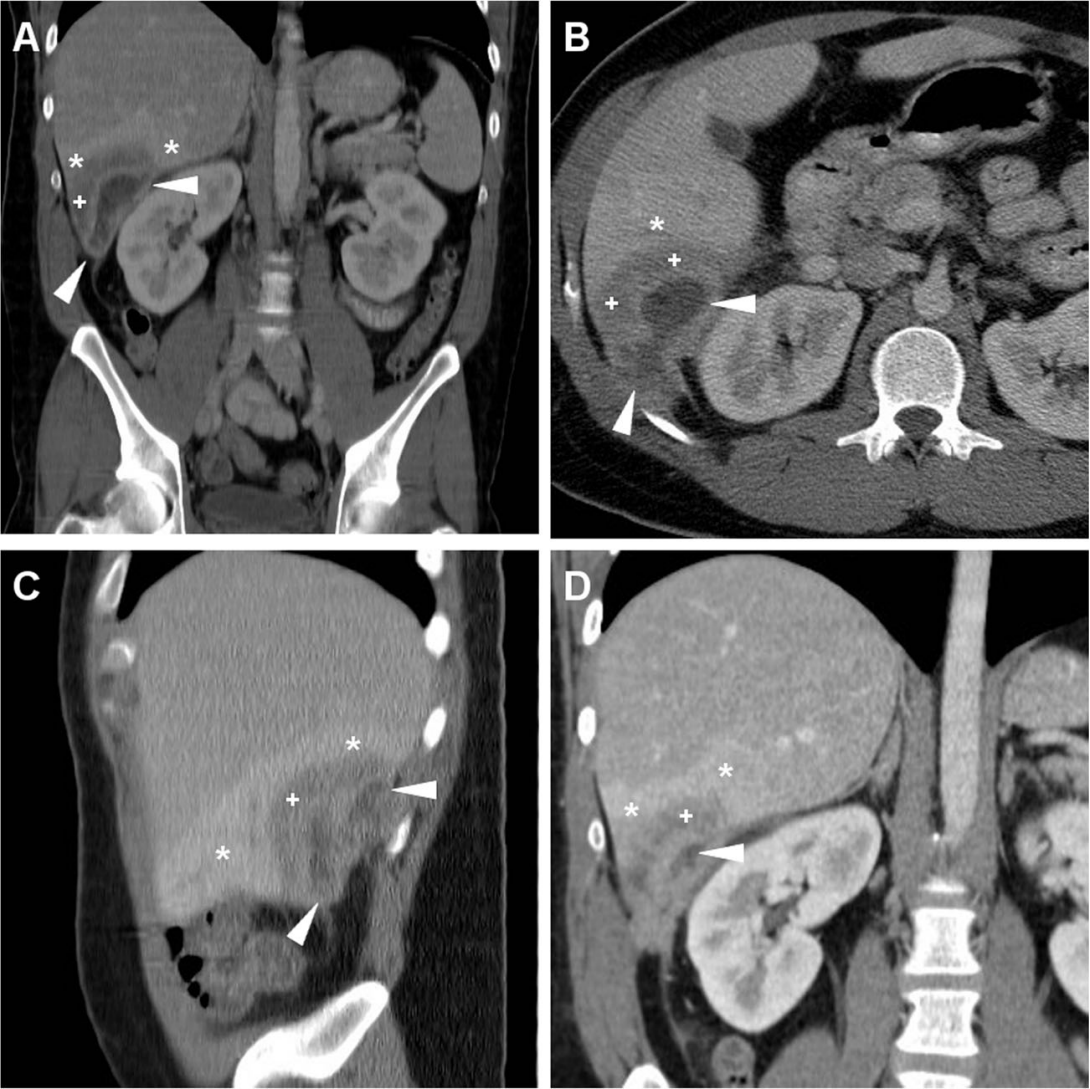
A 24-year-old male presented with mild fever, right lower quadrant pain and absent peritoneal irritation for a week, 3 months after LA for AA. Following ultrasound detection of a poorly defined hypoechoic lesion in the right liver lobe, multiplanar images from contrast-enhanced MDCT (a–c) showed a bilobated abscess in the sixth liver segment, with a strongly enhancing rim (*arrowheads*) and hypodense centre, surrounded by hypoattenuating oedema (+) and hyperenhancing liver parenchyma (*). Percutaneous drainage was not feasible because of the limited size of liquefied content at ultrasound. After antibiotic treatment, the lesion decreased in size at repeated MDCT (d) and ultimately resolved
